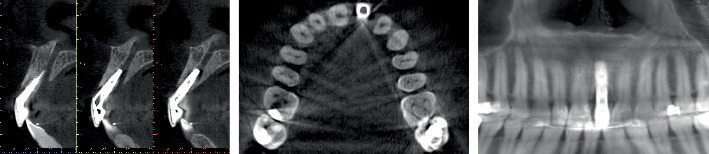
Baseline radiological assessment. Tangential, axial, and panoramic CBCT images of the site. The implant was placed in Zone #21 with excessive depth and buccal–palatal angulation, in addition to the apex projected in the nasopalatine canal, compromising the buccal and palatal plates.
